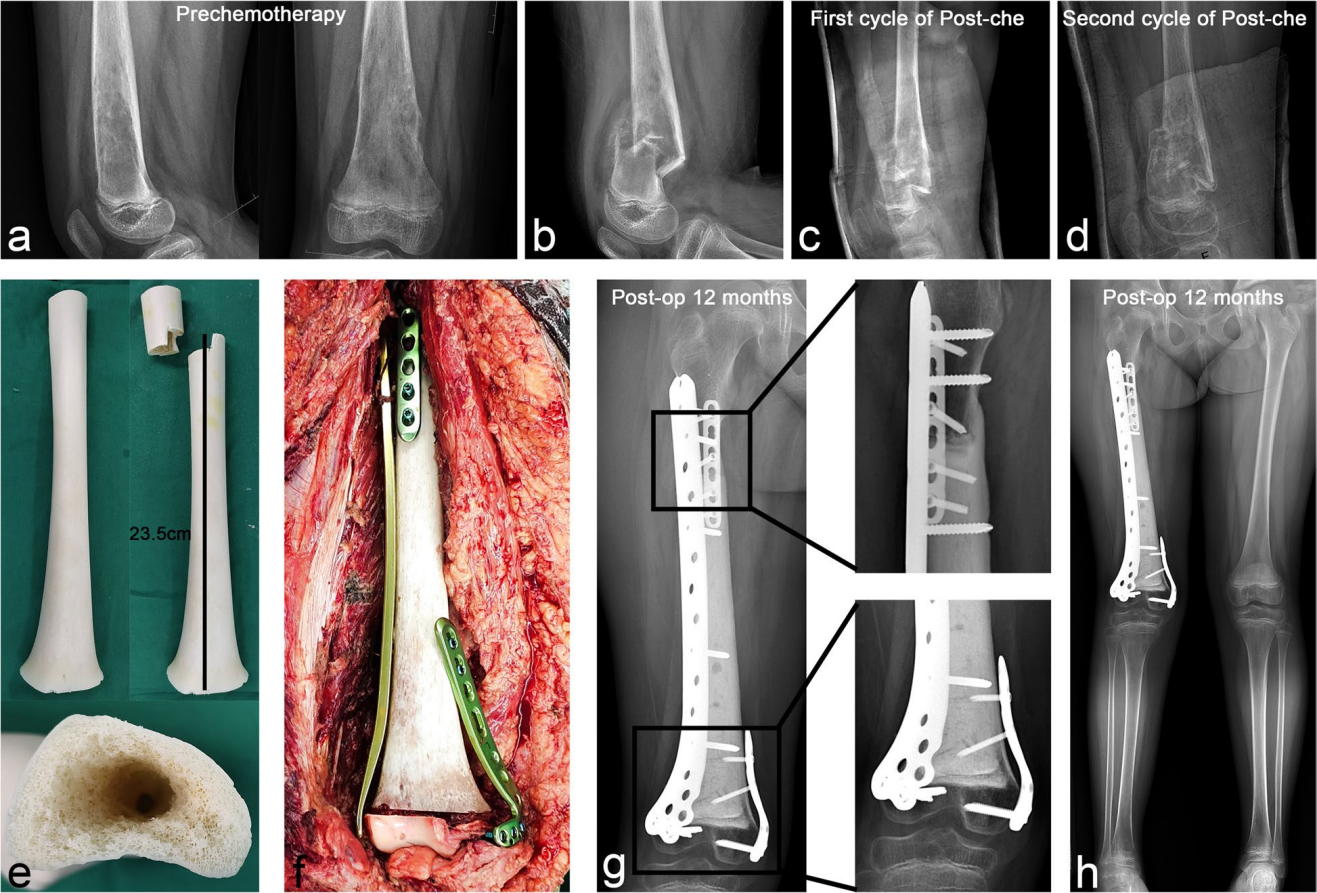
Case 24. A case of stage IIB osteosarcoma in distal femur with pathological fracture. a–d Anteroposterior and lateral X-rays before and after neoadjuvant chemotherapy showed significant bone repair at the fracture site during chemotherapy. e Multidimensional osteotomy was performed at the diaphysis of the allograft to enlarge the host–donor junction surface and empty the medullary cavity for cement filling. f The allograft was fixed to the host laterally with a plate spanning the full length of the allograft and was assisted by a short plate anteriorly to the proximal host–donor junction and medially to the distal host–donor junction, respectively. g The postoperative X-ray at 12 months showed that the host–donor junction of the diaphysis and metaphysis had healed, and h the lower limbs were basically equal in length and had normal force lines
